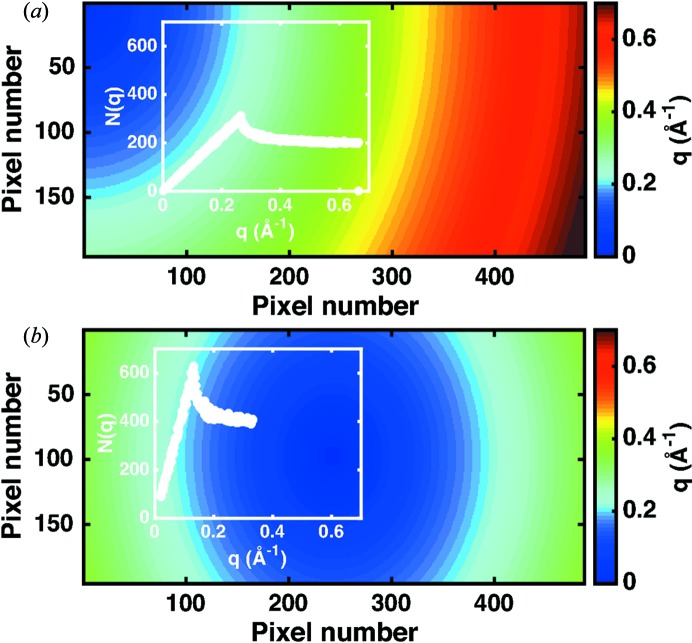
Measurement geometry and the number of pixels per *q* bin. Assignment of the pixels in two dimensions to *q* bins for different positions of the beam centre on a PILATUS 100K detector, with an X-ray energy of 17.4 keV and a sample–detector distance of 1.11 m. (*a*) Configuration with the beam centre aligned on the top left corner of the detector. (*b*) Setup with the beam centred on the detector. The insets show the resulting pixels per *q* bin *N*(*q*). Further beam centre positions are depicted in Fig. S4.
